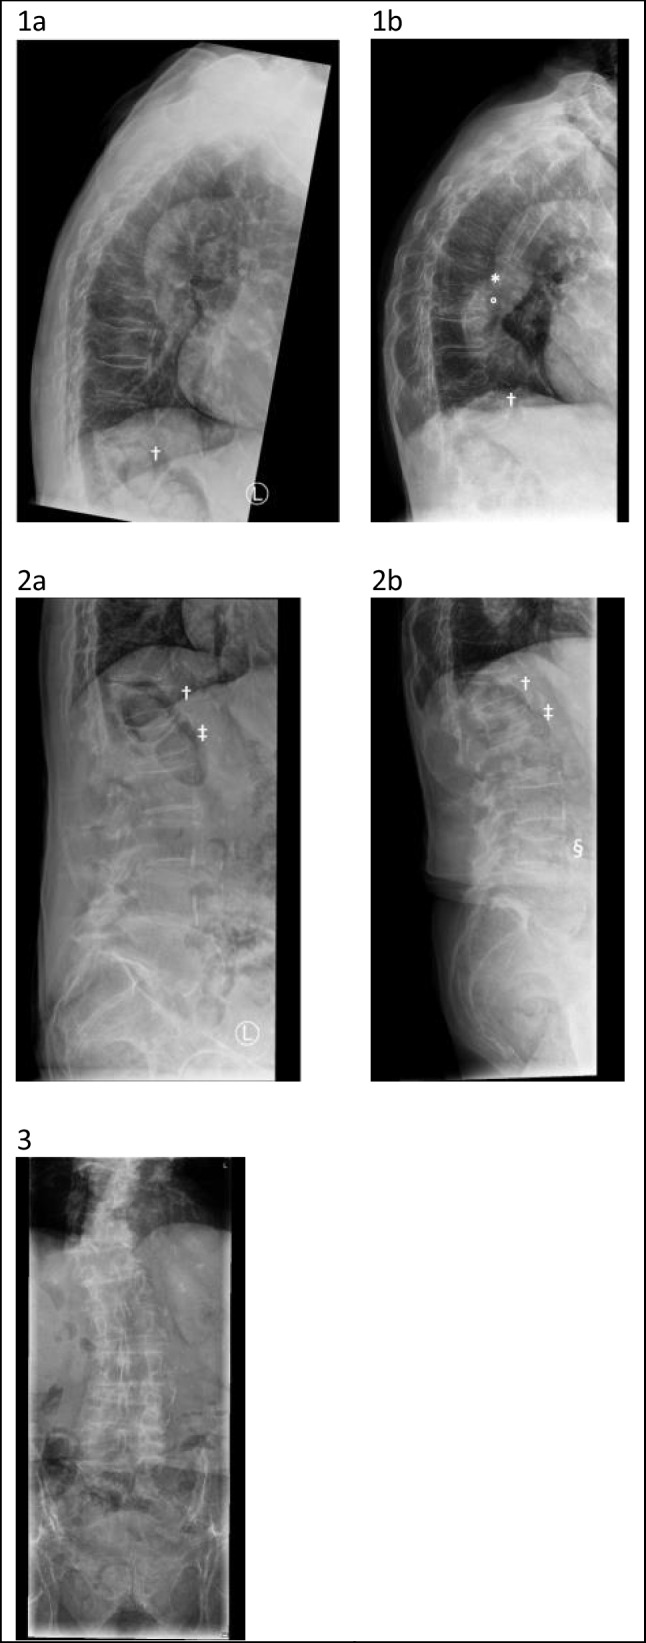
X-rays of the thoracolumbar before and after treatment with romosozumab. Thoracic spine before (1a) and after (1b) treatment with romosozumab. Lumbar spine before (2a) and after (2b) treatment with romosozumab. Thoracolumbar scoliosis (3). * = fracture Th8. ° = fracture Th9. † = fracture Th12. ‡ = fracture L1. § = Fracture L4
